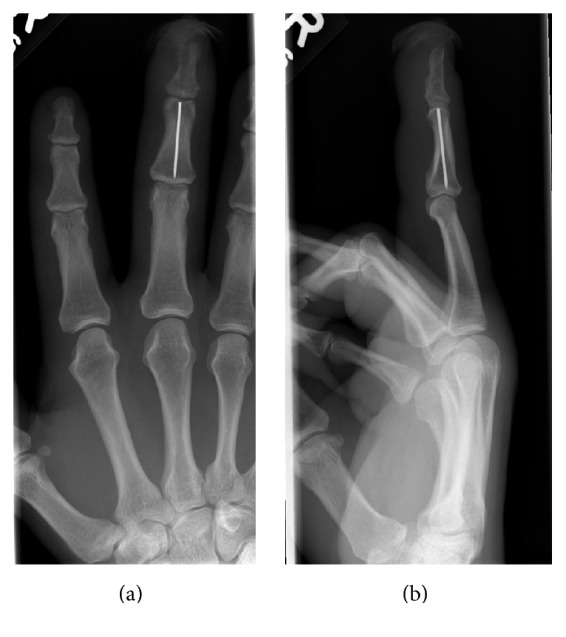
X-rays showing retained Kirschner wire in the right middle finger phalanx.
